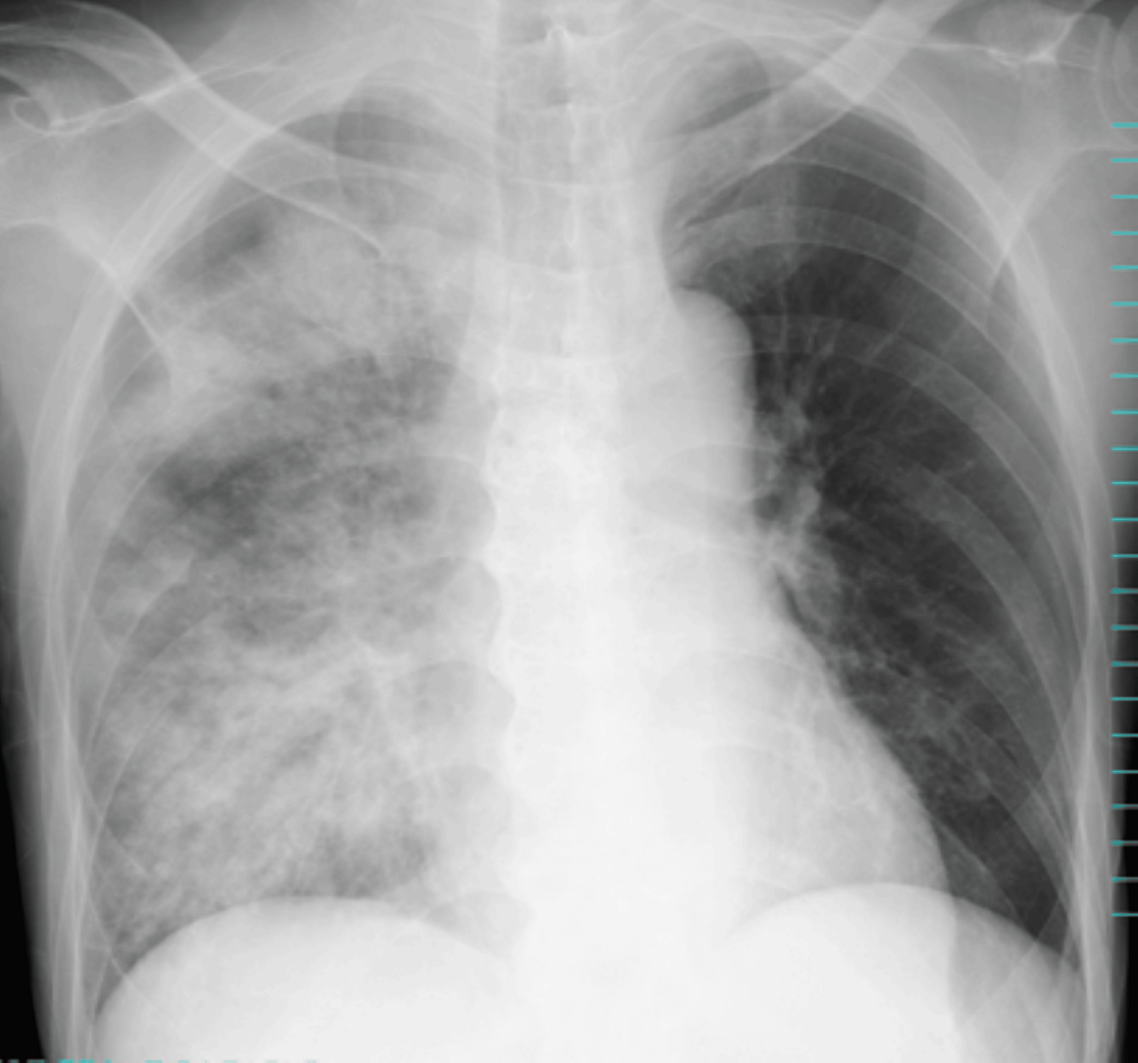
Chest X-ray on day x + 1 at 6:12 a.m. showing diffuse right lung opacity
